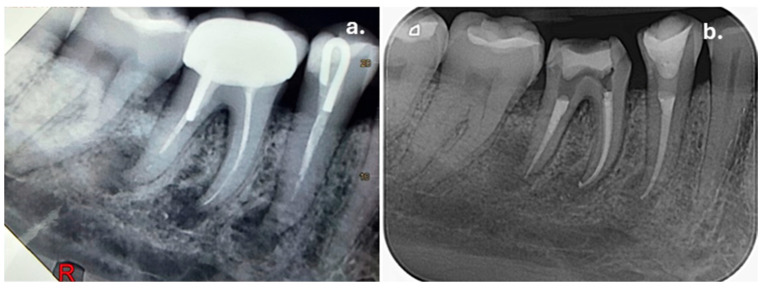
(a) Preoperative periapical radiograph of the mandibular right posterior region, showing periapical radiolucencies associated with teeth 45 and 46 prior to endodontic retreatment. The PAI score for both lesions was estimated as 5; (b) Post-obturation periapical radiograph of teeth 45 and 46.
